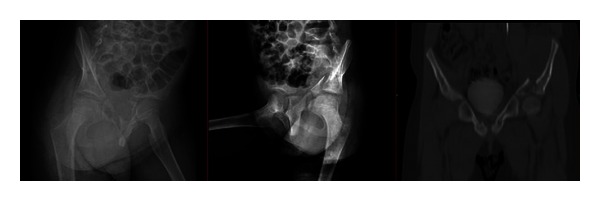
Preoperative iliac oblique and obturator oblique X-ray and pelvic CT.
